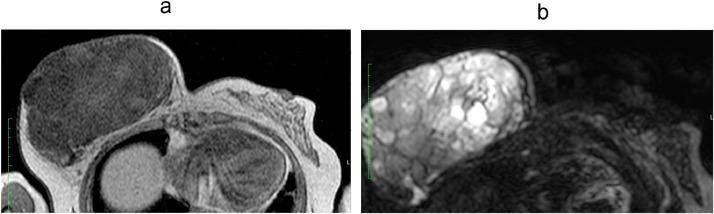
There is a huge contrast enhancing lesion with a circumscribed margin measuring 17.4 × 10.2 × 18 cm (TSxAPxCC) occupying the right breast. It appears isointense on T1-weighted image (Left) with areas of hemorrhage and heterogeneous signal on T2-weighted image (Right) with areas of cystic change.
